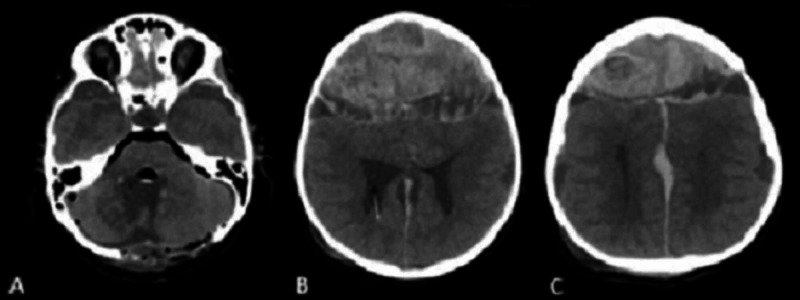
Post-operative non-contrast brain CT scan, axial view A: Gross total tumor resection and pneumocephalus. B: Acute bifrontal epidural hematoma. C: Interhemispheric hemorrhage.
